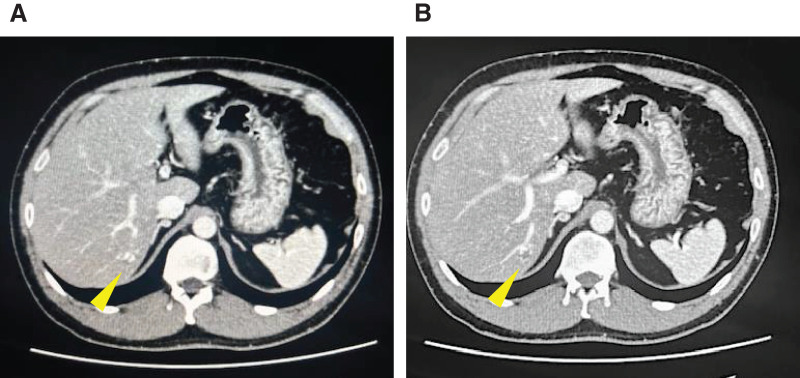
Preoperative contrast-enhanced CT scan. (A, B) A 22-mm nodule (yellow arrow) was detected in hepatic segment 6, with peripheral enhancement in the arterial phase (A) and relatively homogeneous enhancement in the delayed phase (B).
